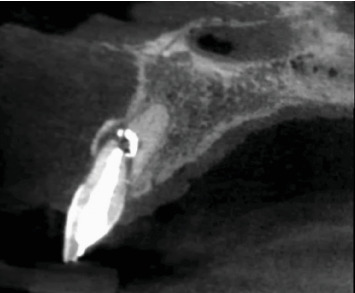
The sagittal CBCT section at 20 years of Tooth 2.1 shows greater displacement of the coronal fragment. The radiolucent area between the fragments has increased, reducing support for the coronal fragment.
